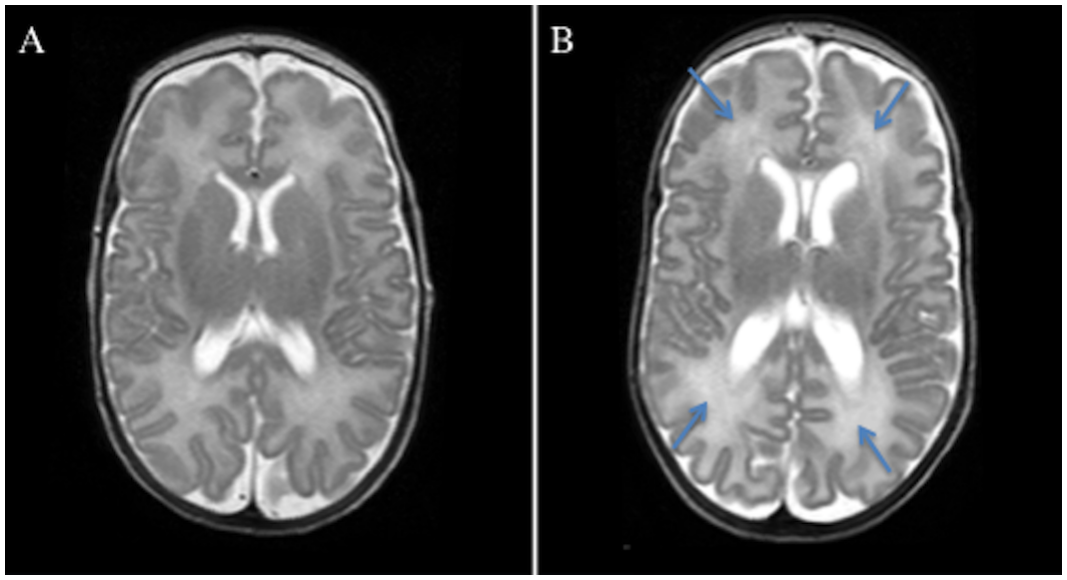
Axial T2-weighted MR images. Normal white and gray matter (A) and diffuse excessive high signal intensity (DEHSI) in the frontal and occipital white matter bilaterally (B). Blue arrows indicate presence of DEHSI.
